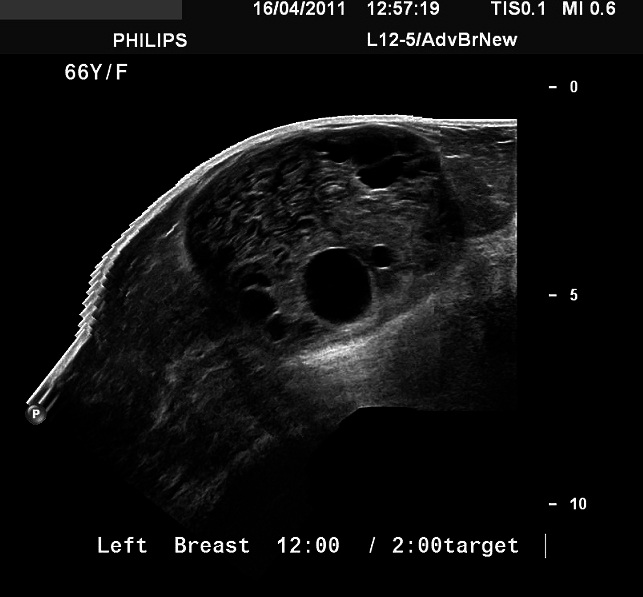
Panoramic ultrasound image of the left breast mass
